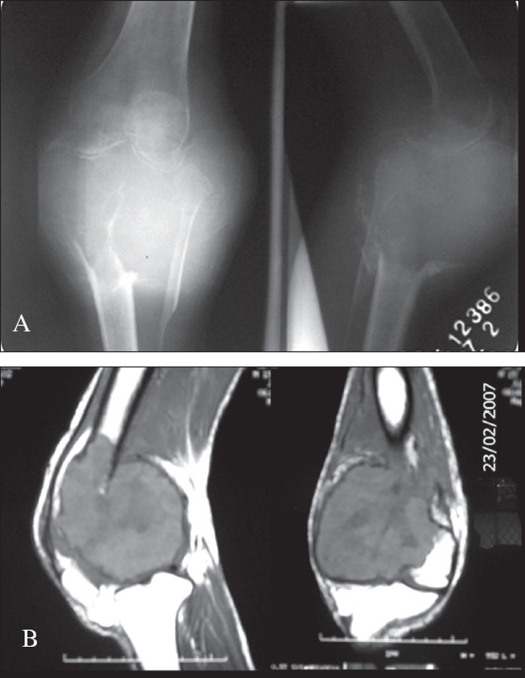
X-ray showing involvement of proximal tibia A) and MRI showing distal femoral involvement B) with tumors with gross cortical destruction and large soft tissue component (in two different patients)
